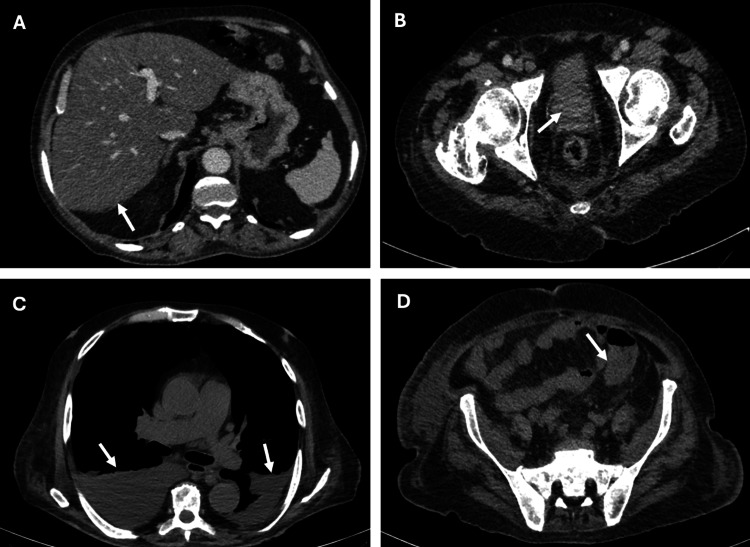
CT scans A) Previous CT abdomen: diffuse liver steatosis (arrow). B) Previous CT-abdomen: prostate malignancy (arrow). C) Current CT angiogram: moderate-sized bilateral pleural effusions, larger on the right (arrows). D) Current CT angiogram: fluid-filled rectosigmoid (arrow) with no convincing evidence of contrast blush to suggest active haemorrhage. There is some apparent wall irregularity of the distal sigmoid and the rectum.
